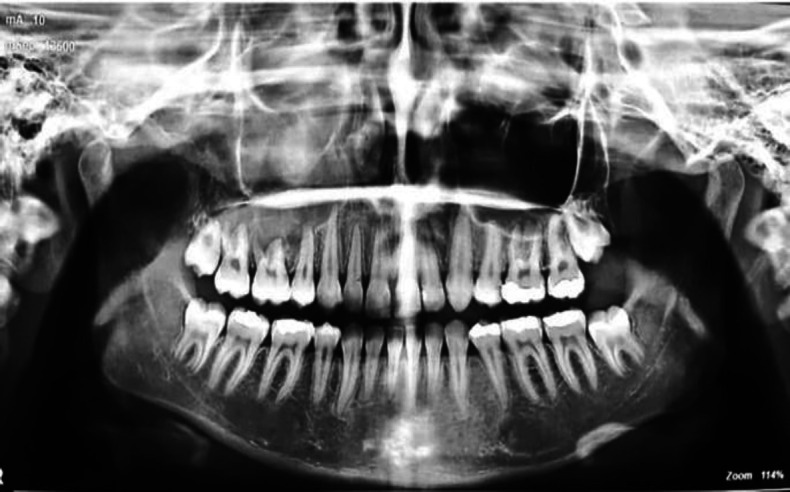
Panoramic radiograph showing opacification in the right maxillary sinus with root resorption of teeth
